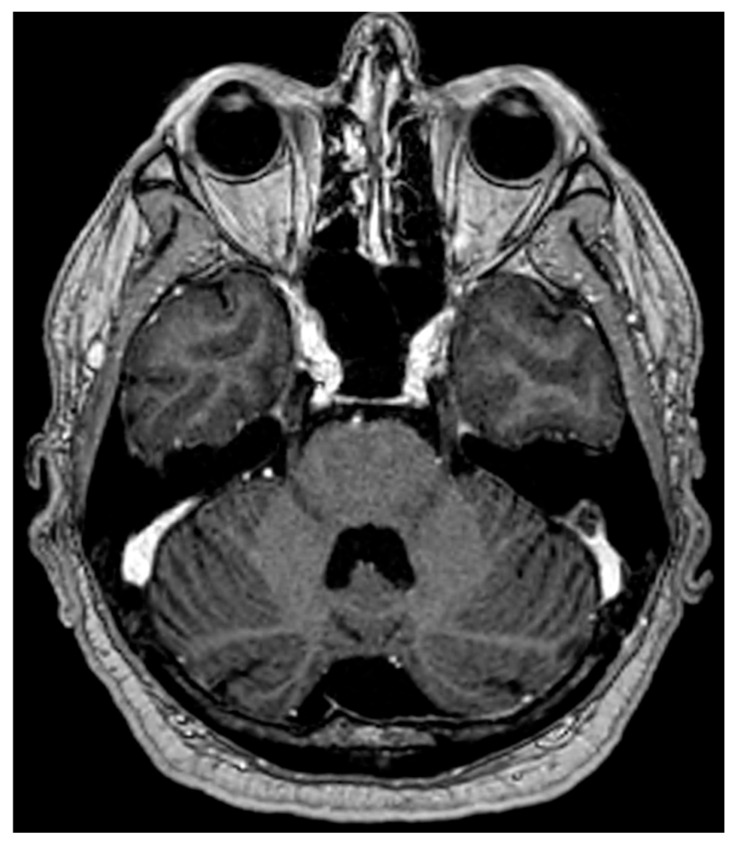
In the same patient shown in Figure 1, a post-contrast T1W MRI is proposed, focused on the plane of the cisternal TN (same plane as in Figure 1c), without contrast enhancement.
